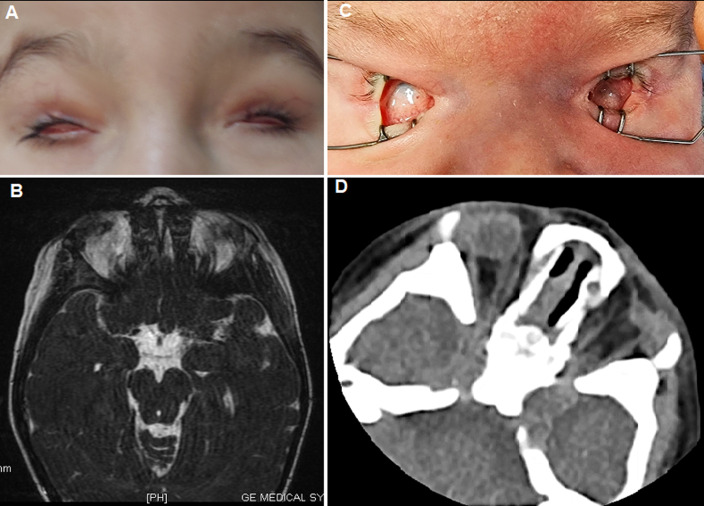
A) eye photograph of the six-year-old sister showing bilateral severe microphthalmia; B) T2-weighted MR scan of the sister revealing rudimentary severely hypoplastic eyeballs and the presence of amorphous tissue and structures; C) clinical appearance of the bilateral severe microphthalmia in the newborn; D) coronal FLAIR sequence of the newborn demonstrating rudimentary severely hypoplastic eyeballs and vestigial optic nerves
